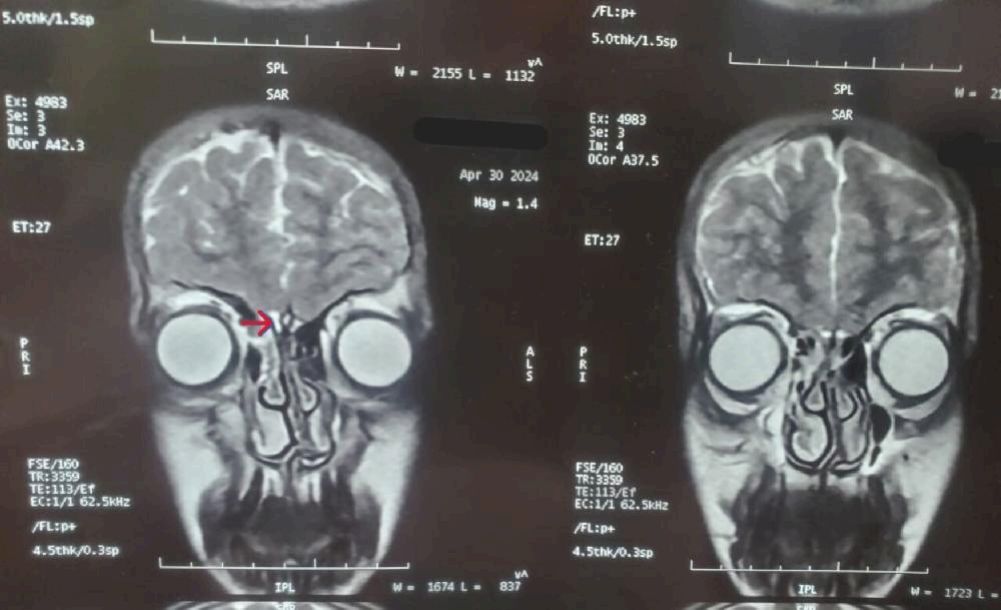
Coronal section of magnetic resonance imaging brain shows normal olfactory bulb (red arrowhead), which is bilateral symmetrical.
